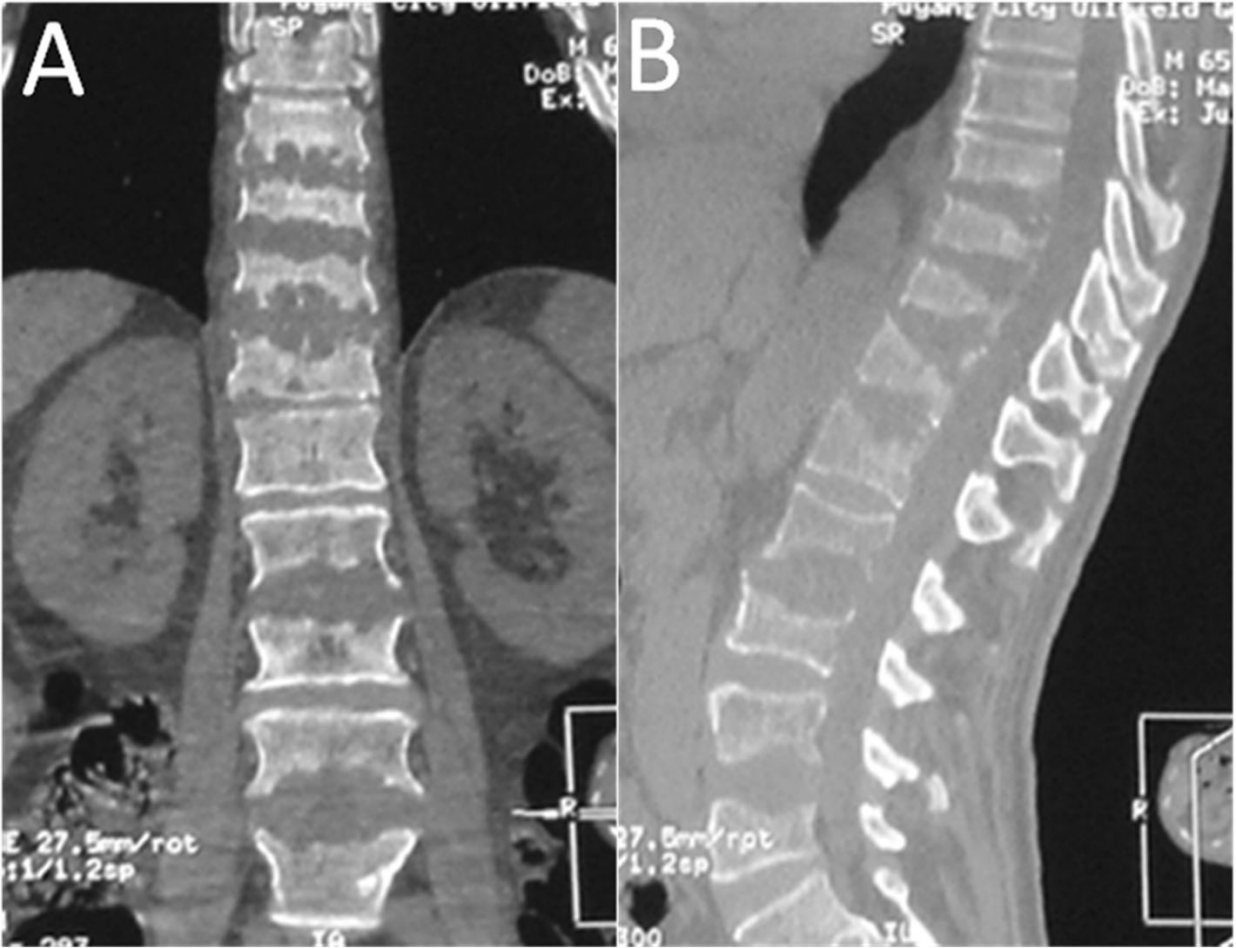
A 56-year-old man was diagnosed as having T9-12/L2-3/L4-5 fungal spondylodiscitis. The CT scan showed the endplates were grossly destroyed
